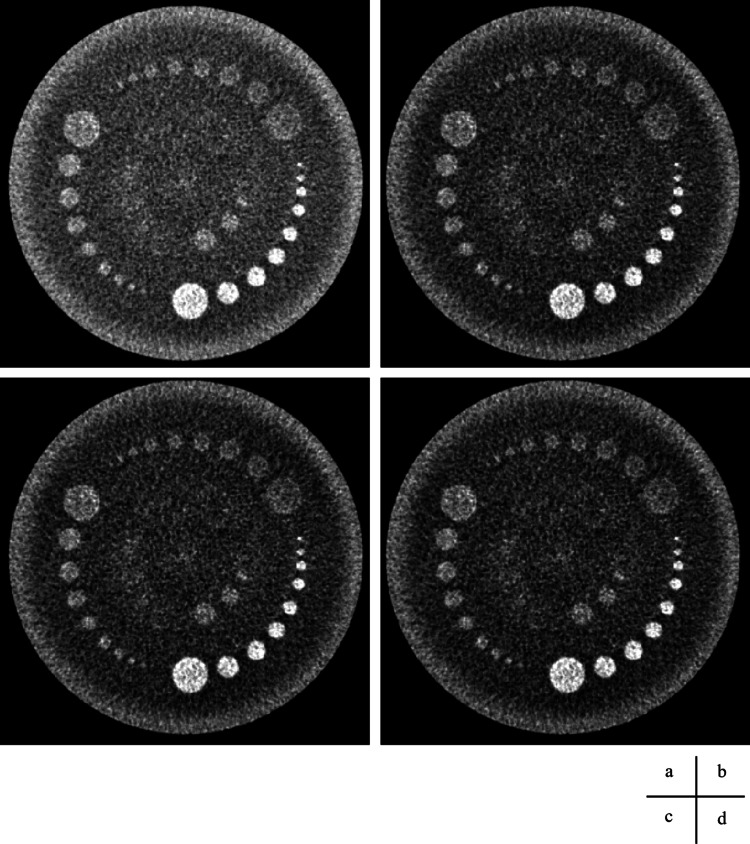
Helical scan. Computed tomography image and processed images using square transformations, displayed with a narrower window width to enhance contrast. (a) Original image: window level (WL) = 50 Hounsfield units (HU); window width (WW) = 20 HU. (b) Square−5 image: WL = 2425 HU; WW = 1800 HU. (c) Square image: WL = 2900 HU; WW = 2000 HU. (d) Square+5 image: WL = 3425 HU; WW = 2200 HU.
